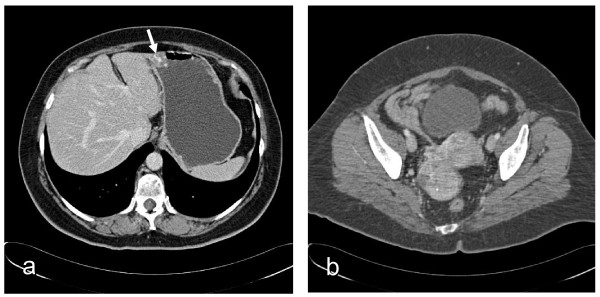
CT appearance of gastric and ovarian neoplasms: 30-mm solid mass with minute calcifications located between the anterior wall of corpus and gastric antrum (a, arrow). Pelvic mass of 65×45×60 mm on right adnexa suggestive of mixed cystic-solid ovarian tumor (b).
